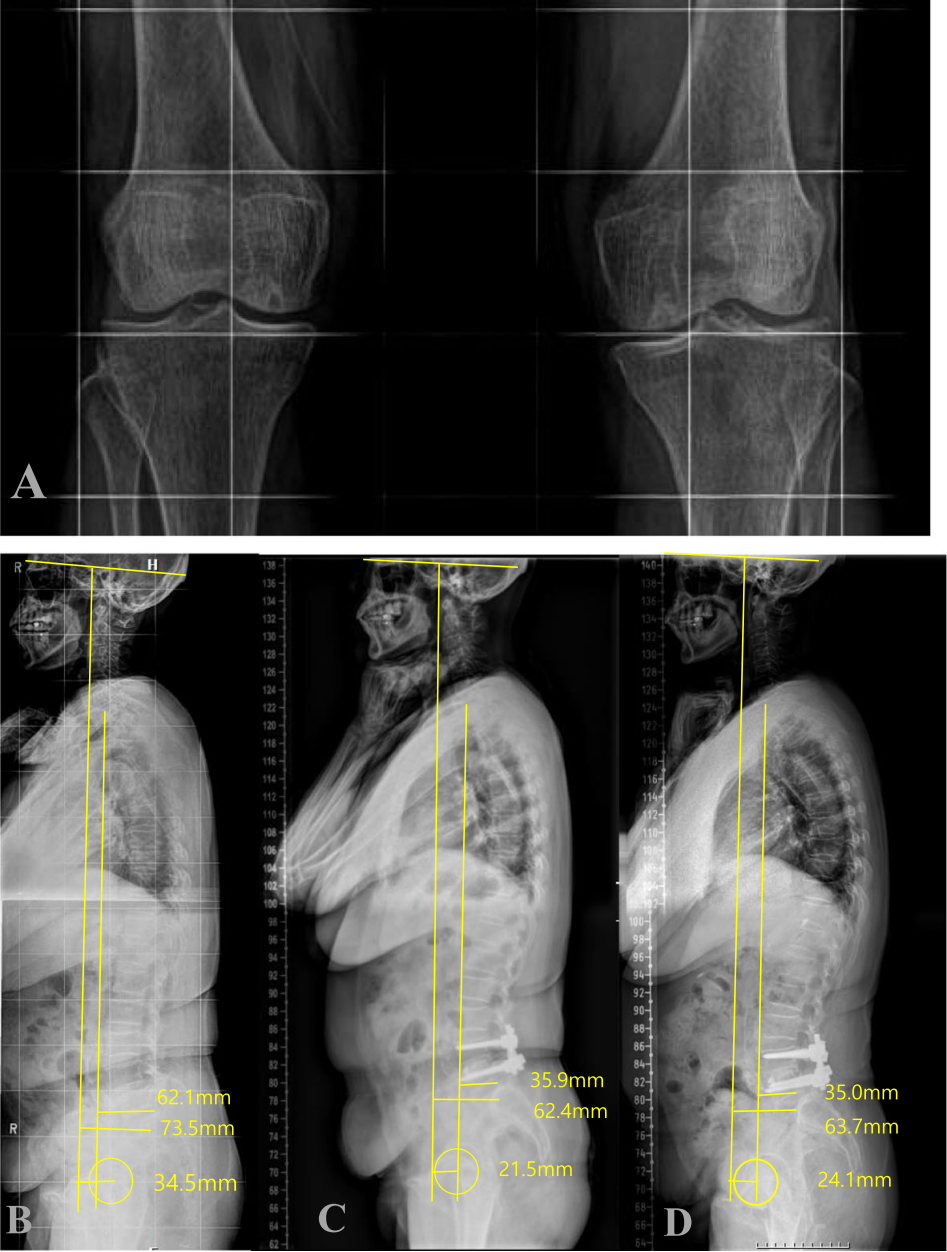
A case of 64-year old female, who underwent L4-L5 instrumented fusion. a Preoperative standing knee AP radiograph shows mild (KLG 1) knee OA. Whole spine standing lateral radiographs taken at b the preoperative, c the postoperative 1 month, and d the postoperative 3-year follow-up show the stable maintenance of postoperatively achieved improvement in radiographic sagittal balance
